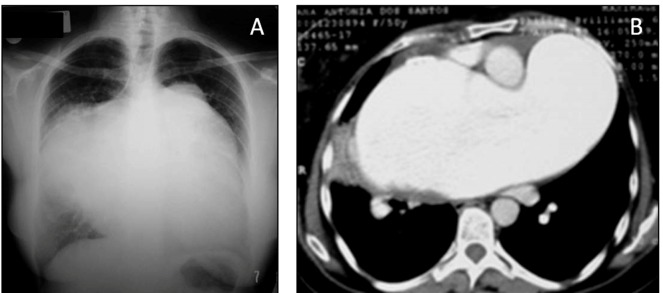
Chest X-ray (A) and computed tomography scan
